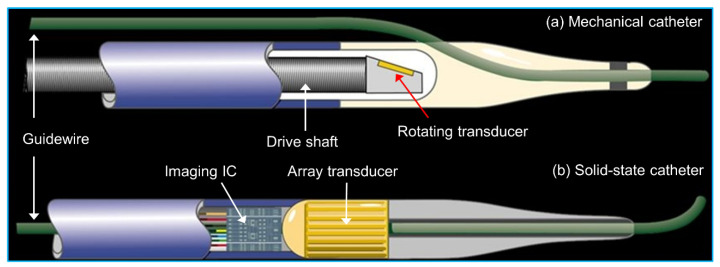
Two different types of IVUS catheters: (a) mechanical/rotational catheter with a single-element ultrasound transducer; (b) solid-state catheter with a phased-array ultrasound transducer. Reprinted with permission. (Figure courtesy: Boston Scientific, Inc., Marlborough, MA, USA, https://www.bostonscientific.com, accessed on 6 January 2021).
